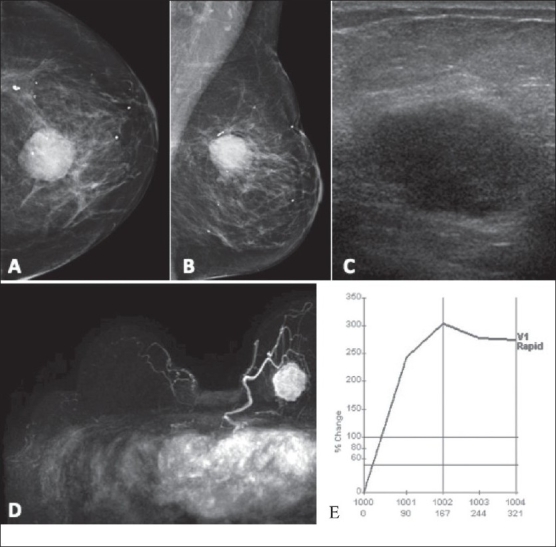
A 66-year-old woman with a remote history of bilateral breast cancer and radiation therapy presented with a new rapidly growing mass. Craniocaudal (A) and mediolateral oblique (B) mammograms show a round mass. USG (C) demonstrates a hypoechoic oval mass with no posterior enhancement, classified as BI-RADS 5. Biopsy showed sarcoma. Pre-operative MRI (D) shows a round mass with a lobulated margin, with no chest wall invasion. Kinetic assessment (E) demonstrates rapid initial enhancement followed by washout in the delayed portion of the curve, classified as BI-RADS 6
